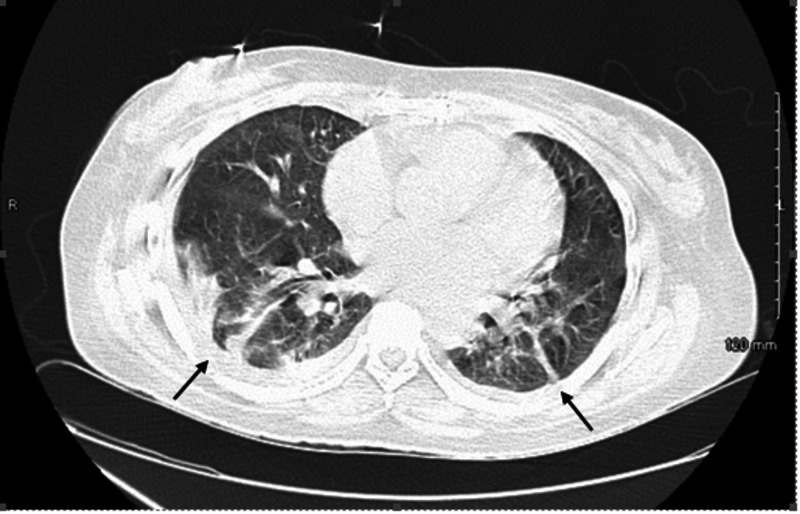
CT chest (axial lung window) showed bilateral pleural effusions and pulmonary infiltrates
